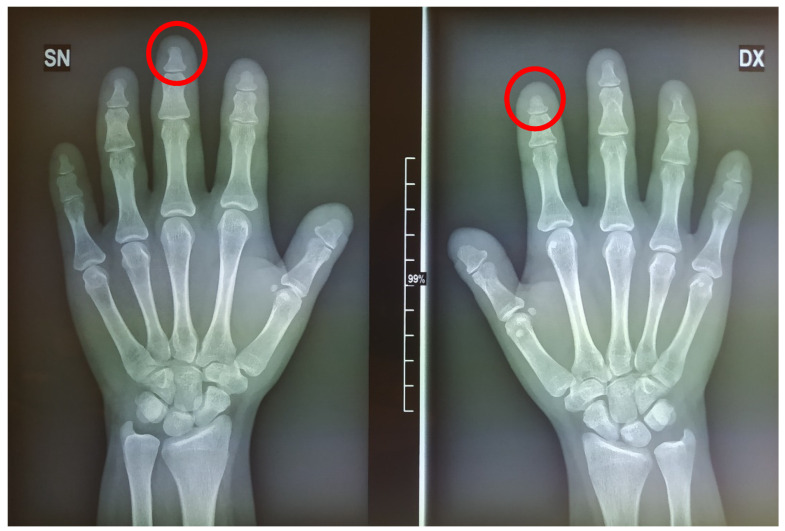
Left- and right-hand X-rays in 2021. Evident short terminal phalanges (red circles marking examples).
